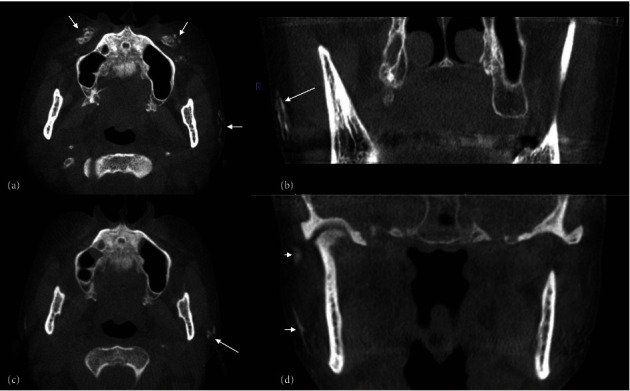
CBCT images of the subdermal CaHA filler (case 2): (a) axial and (b) coronal CBCT images showing multiple, conglomerate high-attenuation entities within the dermal layers anterior to the maxillae and lateral to the masseter muscles; (c) axial and (d) coronal CBCT images after 9 months showing resorption and posterior migration of the filler.
